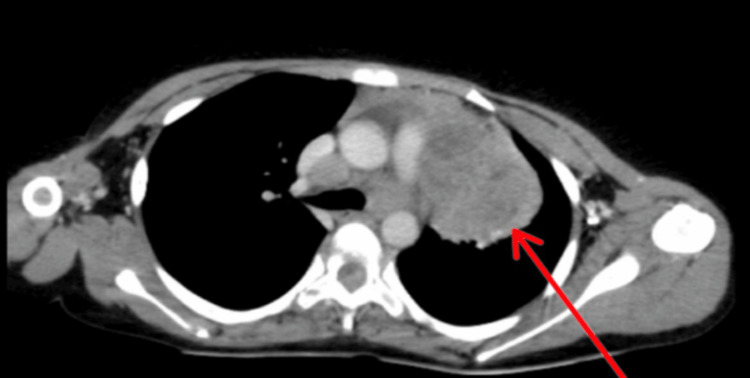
Contrast-enhanced CT showing mediastinal lesion with encasement of the arch of the aorta and bilateral pulmonary arteries.
